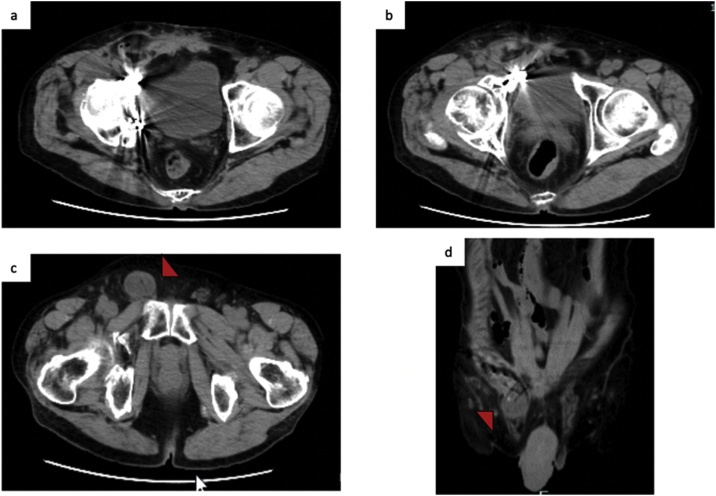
Computed tomography 18 months after pelvic fracture surgery. a, b: The identification of hernia orifice is difficult due to plate artifacts. c, d: The hernia sac reaches the scrotum and small bowel was incarcerated (red triangle).
